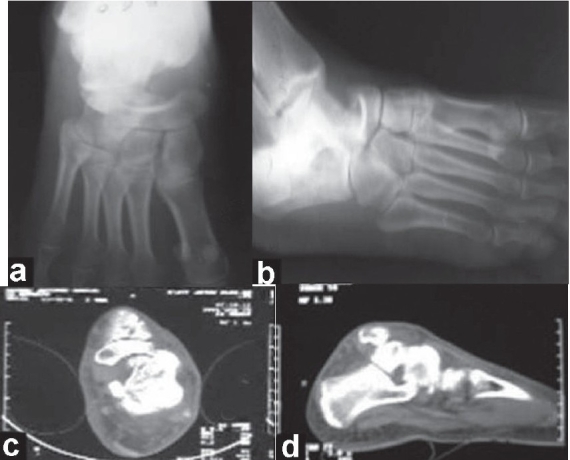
Preoperative radiographs anteroposterior (a); and oblique (b) and CT scan images (c and d) of left foot showing talonanicular dislocation, compression fracture of head of talus, intact calcaneocuboid joint, and a normal subtalar alignment.
